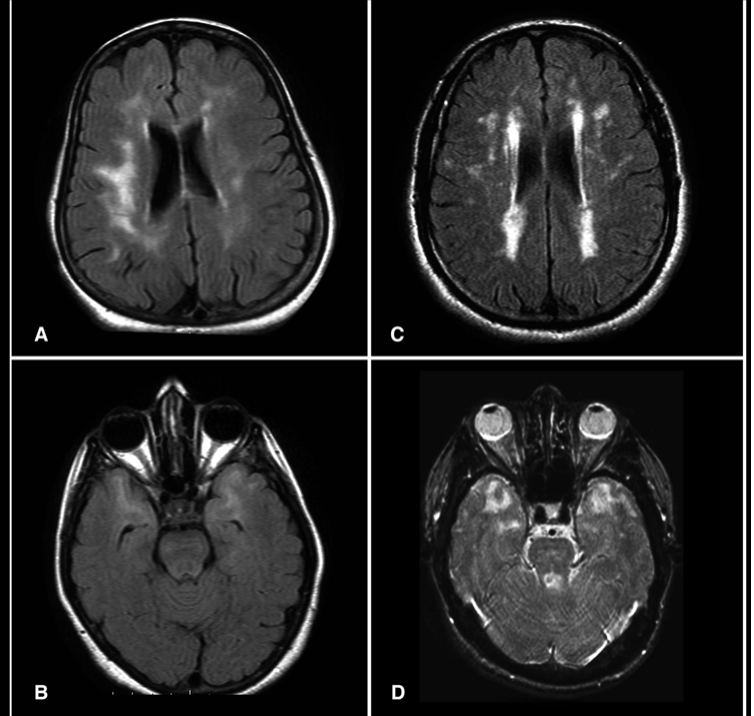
Axial FLAIR (a, b & c) and T2 weighted (d) Brain MRI from patients. The exams in 2a and 2b are from asymptomatic patients with depression. Note temporal lobe lesions even in asymptomatic patients (2b). In Figure 2a and d periventricular diffuse white matter ischemic lesion and multiple lacunar lesions in thalamus, pons and basal ganglia.
